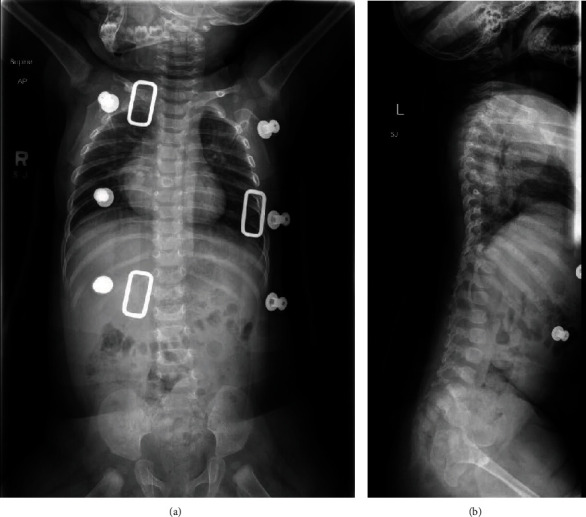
(a, b) Postoperative AP spine and sagittal radiographs with no evidence of residual tumor or further progression of scoliosis.
